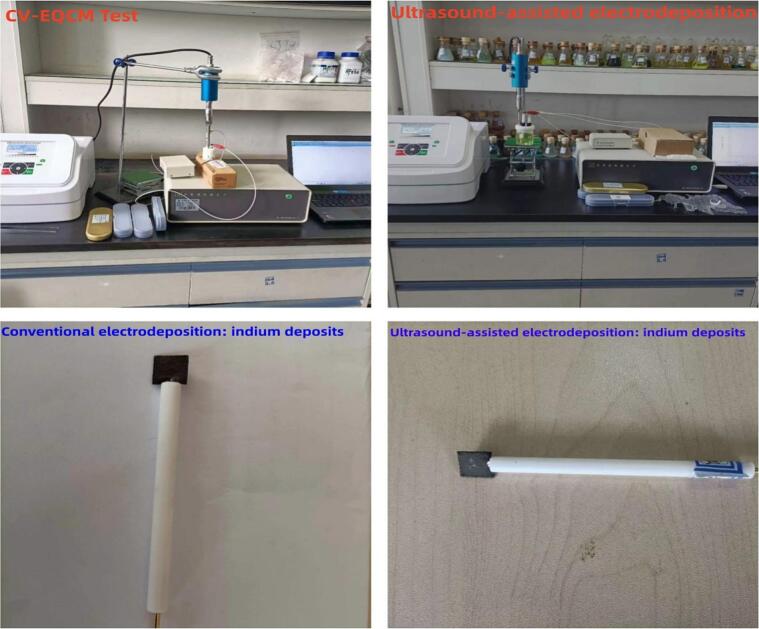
Practical experiments of conventional electrodeposition of indium and ultrasound assisted electrodeposition of indium.
